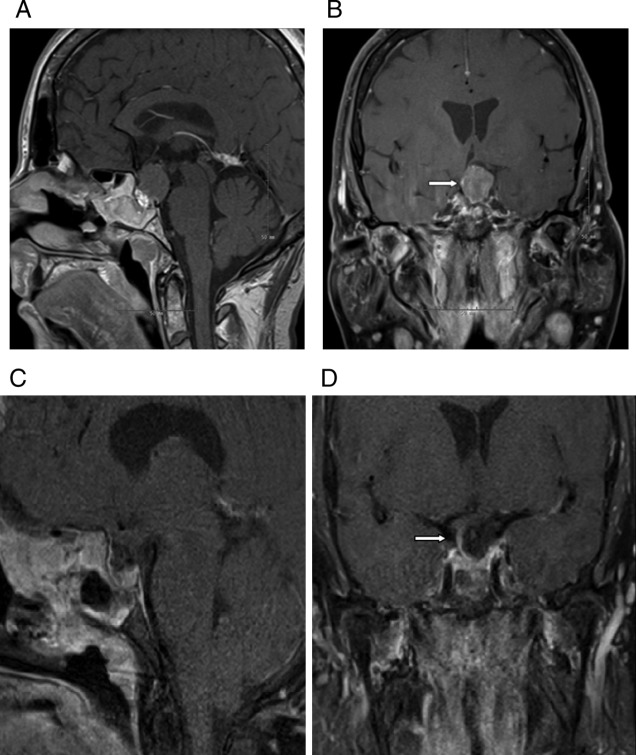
Magentic resonance imaging results. (A and B) T1-weighted, post-gadolinium coronal (A) and sagittal (B) images demonstrating a large pituitary macroadenoma (arrow) present on sellar imaging 6 weeks after the initial surgery; a fat graft (with very high signal intensity) lies in the floor of the sella just anteroinferior to the compressed normal gland (best observed on the coronal view, A). The normal gland, lying in the floor of the enlarged sella, enhances avidly and is compressed by the suprasellar tumor, as best appreciated on the sagittal view. The tumor was suprasellar, above the diaphragm as observed at surgery, and compressed the diaphragm; the infundibulum is compressed and distorted to the right of the tumor and enhances more than the tumor itself (the arrow in B identifies the stalk as it passes to the compressed pituitary in the floor of the sella). (C and D) T1-weighted, post-gadolinium coronal and sagittal images 6 weeks after the second transsphenoidal surgery, with complete resection of the suprasellar, intracranial component of tumor. The infundibulum is now more easily identified, although still deviated to the right (arrow in D). The normal pituitary gland has expanded to fill more of the sella (best appreciated on the sagittal view, C).
